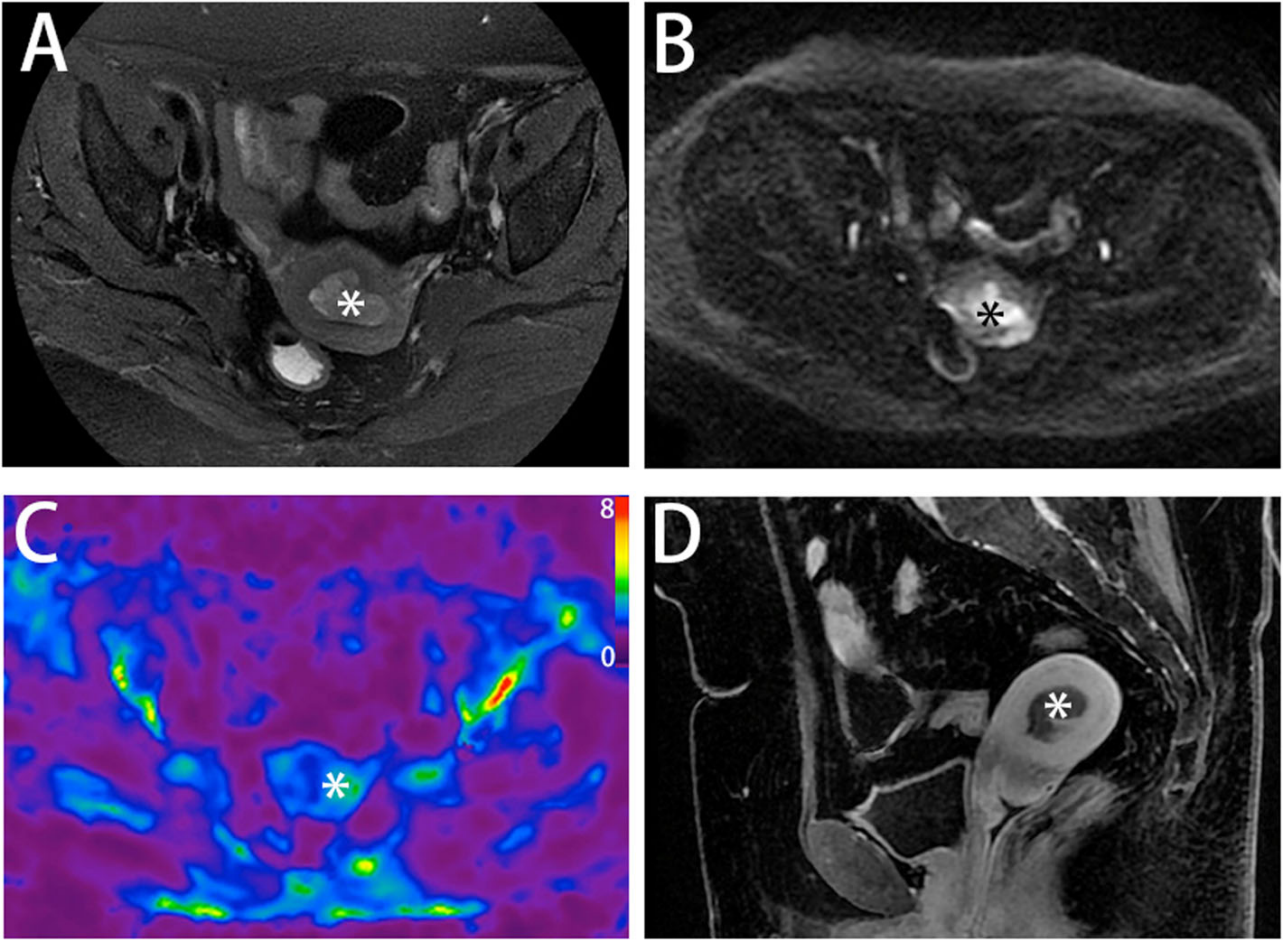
A 35-year-old woman presented with abnormal vaginal bleeding and underwent pelvic MRI and MRE scans. Axial T2-weighted image (A), diffusion-weighted image (B), and sagittal T1-weighted image with contrast (D) showed a mass (2.8×2.7 cm, asterisk) in the uterus. Tumor stiffness was 2.39 kPa measured by 3D MRE elastograms (C) at 60 Hz. Well-differentiated endometrial adenocarcinoma (Type I) with superficial myometrial invasion was surgically confirmed
